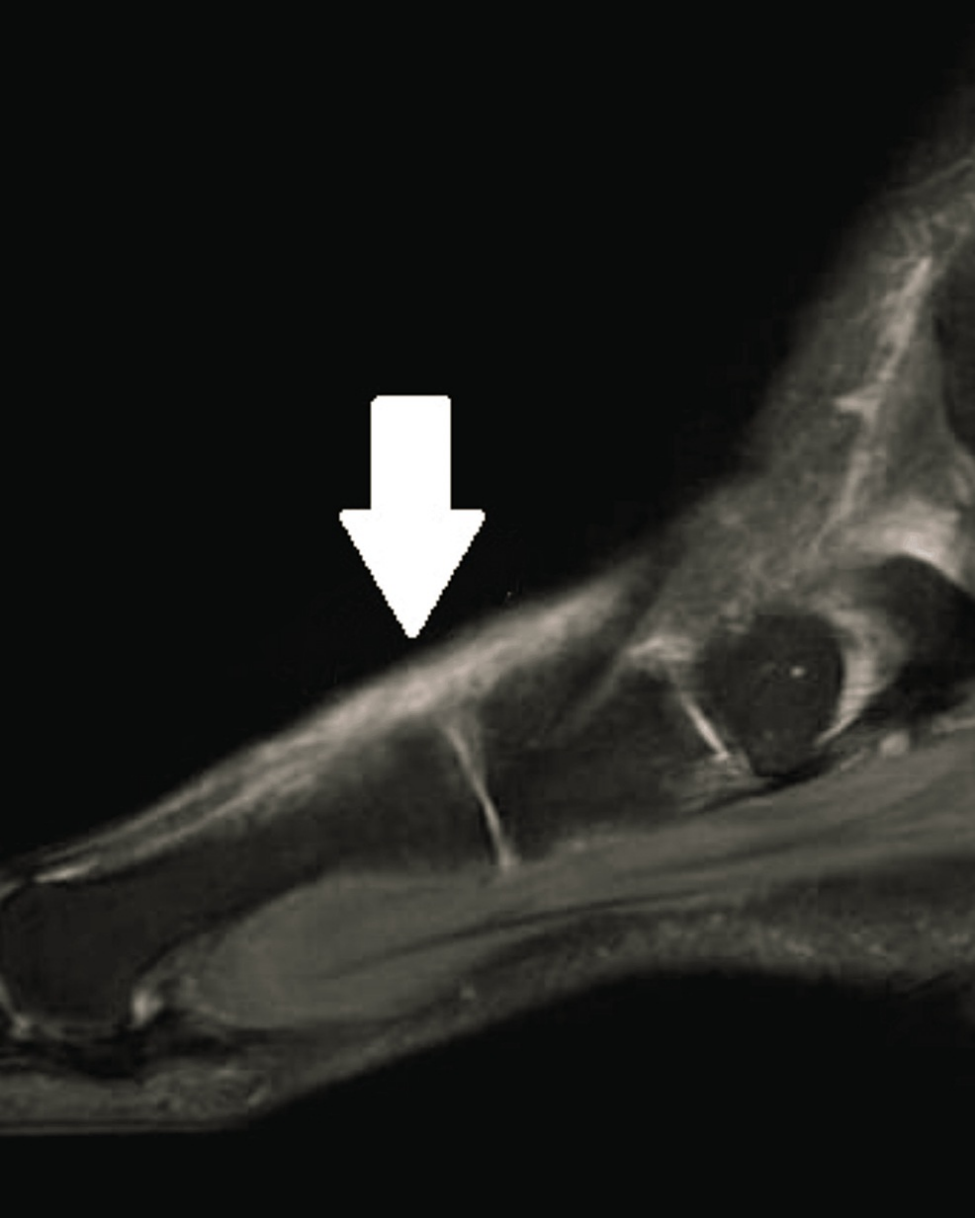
MRI showing extensor hallucis longus rupture
